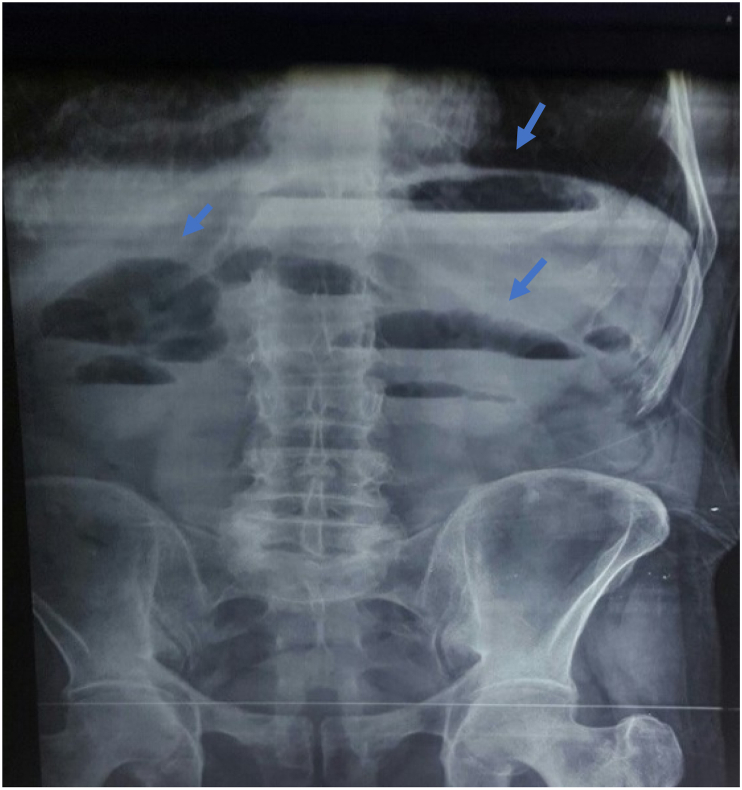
Abdominal X-ray showing air-fluid levels (arrows).
